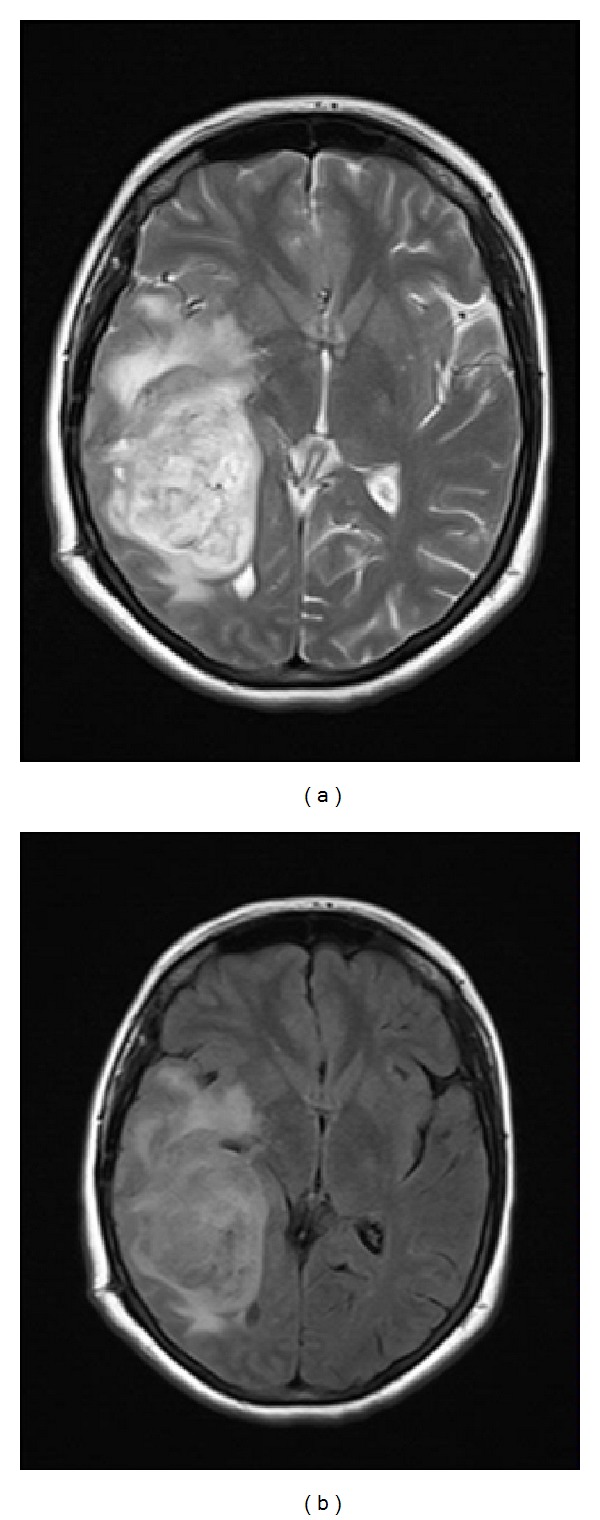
T2 weighted (a) and (b) FLAIR MR images of the lesion before second operation on axial plane showing a recurrent lesion with prominent peripheral edema and minimal midline shift at the same location.
